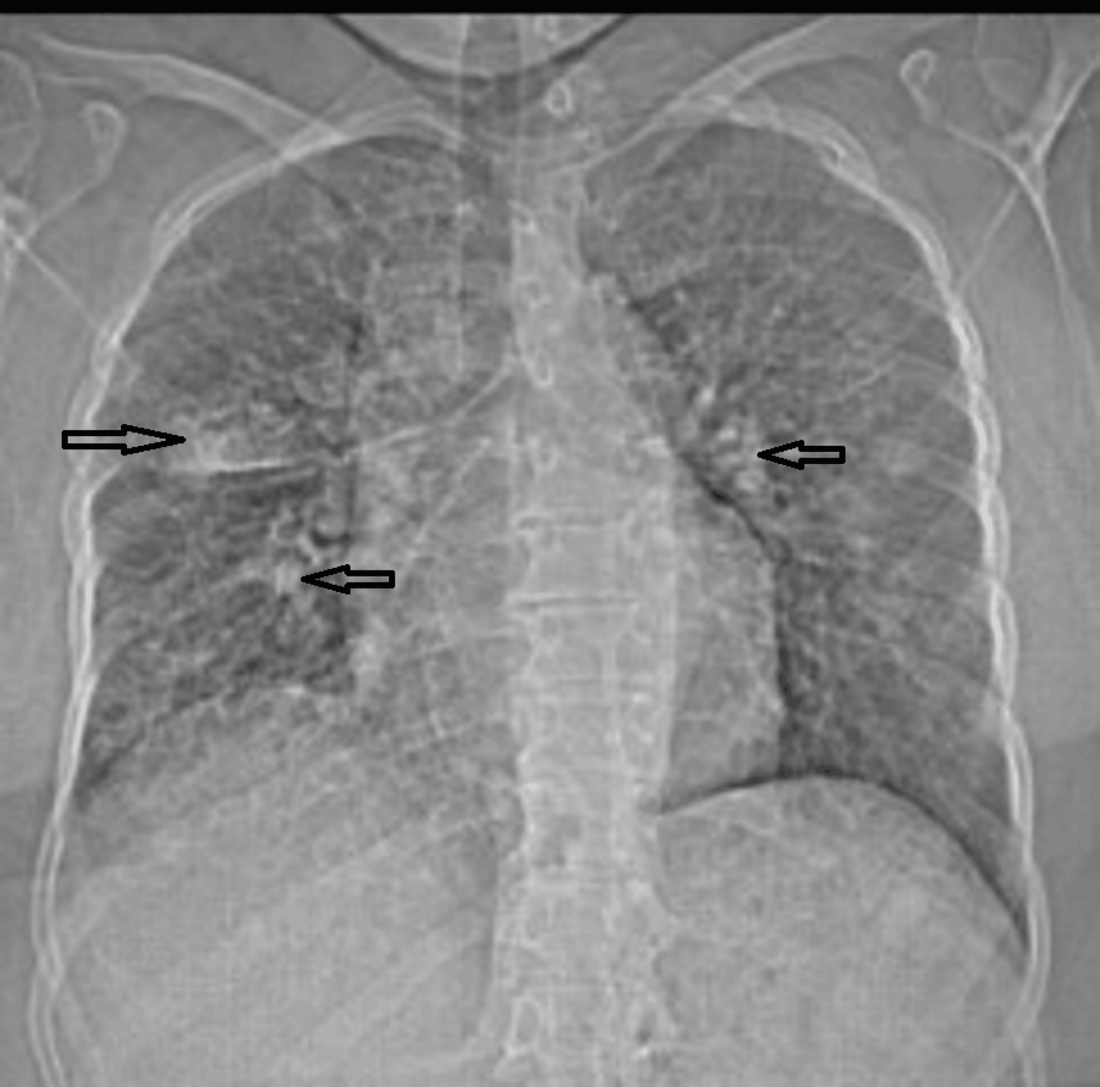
Chest X-ray shows bilateral diffuse ground glass opacities and infiltrates (arrows), suspicious for pneumonia.
